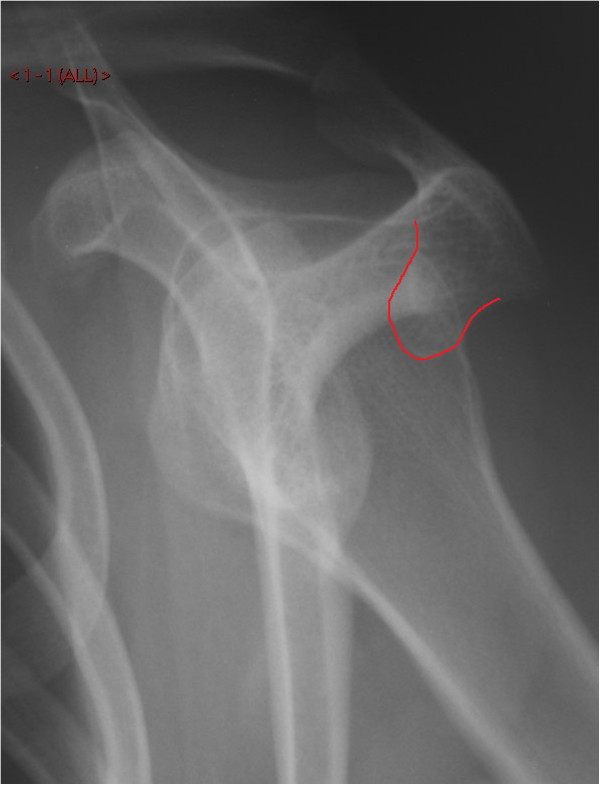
A lateral (scapular Y-view) radiograph of the shoulder joint demonstrating the exostosis protruding into the subacromial space which is outlined by the red line.
